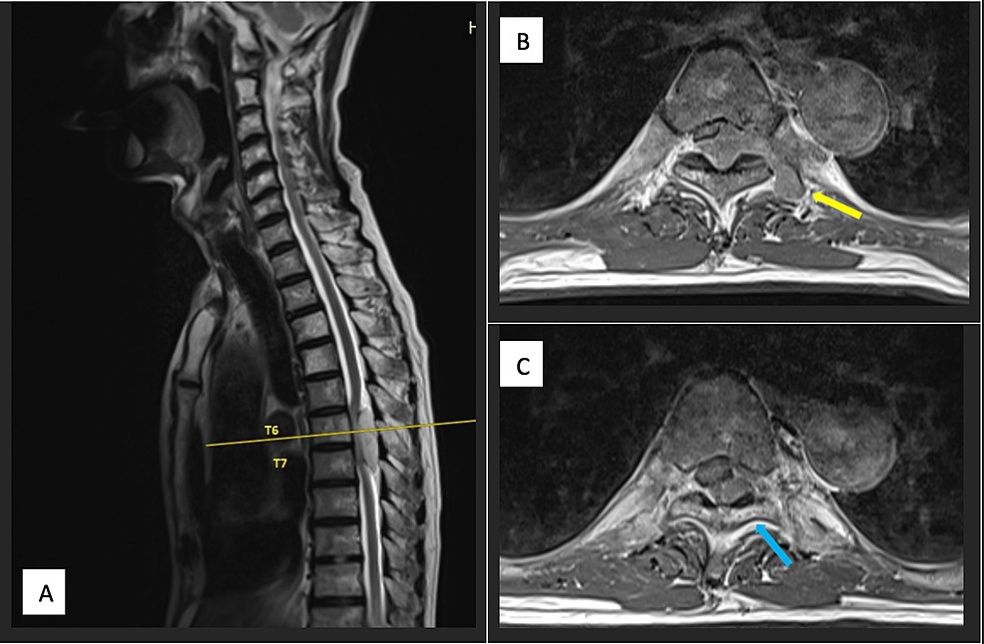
(A) Sagittal T2-weighted MRI showing a soft tissue tumour spanning the T6 to T7 epidural space. (B) Axial MRI image at T6 showing tumour exerting mass effect on the spinal cord with left neuroforamen extension forming a “dumbbell-like” tumour (yellow arrow). (C) Axial cut at T7 showing tumour extension (blue arrow).
